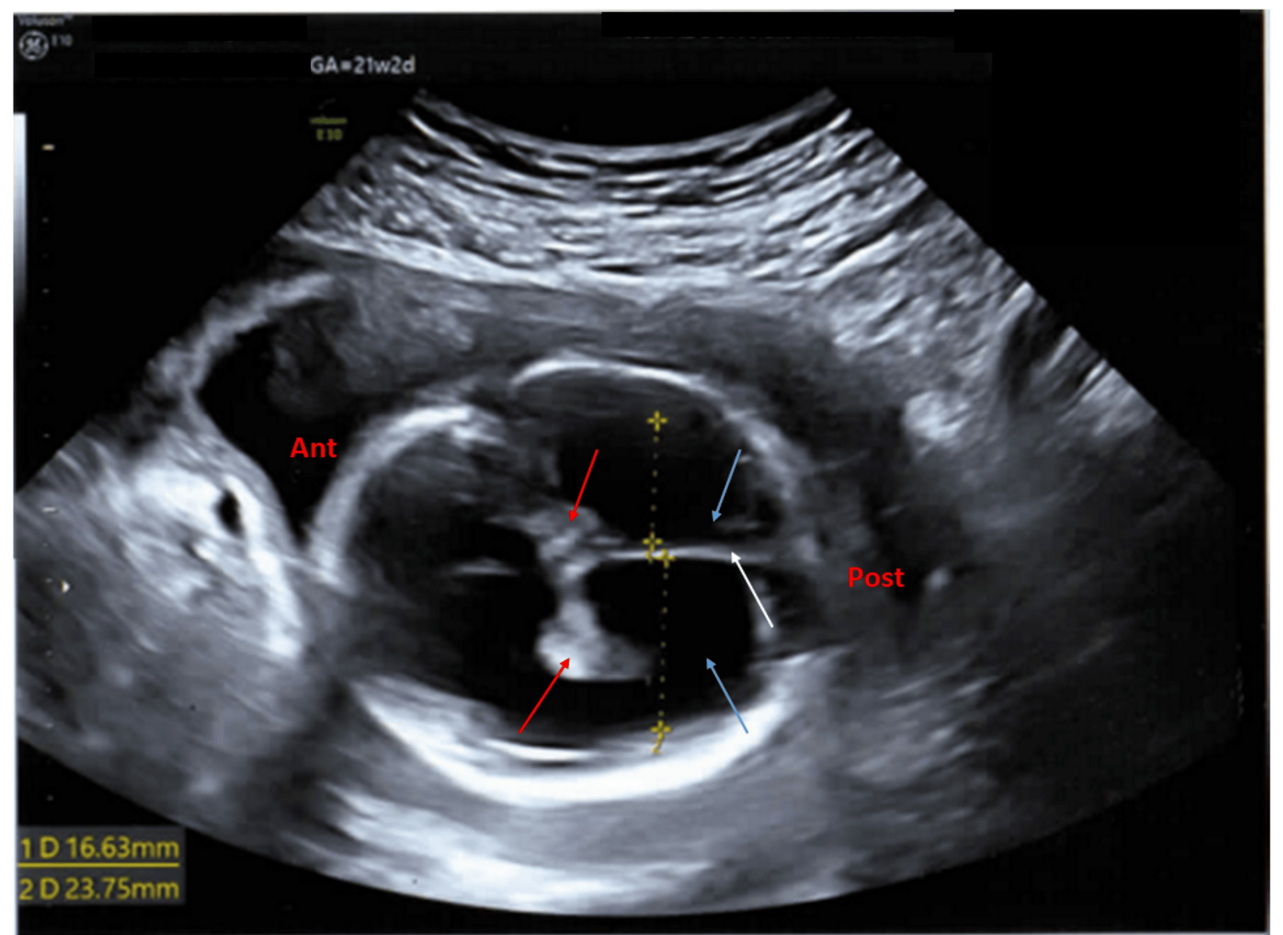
Antenatal ultrasound of the fetus in the second pregnancy showing cerebral ventriculomegaly Antenatal ultrasound of the fetus shows a transverse view of the fetal skull (ant: anterior side, post: posterior side) around 21 weeks 2 days gestation, with dilated cerebral ventricles (blue arrows) (measuring 16.63 mm and 23.75 mm in diameter) showing a dangling choroid plexus (red arrows); the falx cerebri is normal (white arrow).
